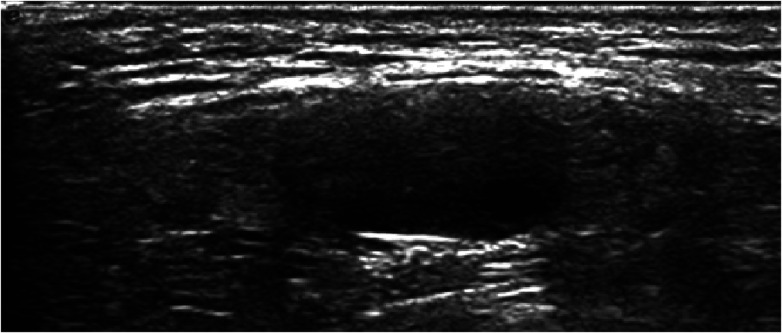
Ovotestis on ultrasonography.
